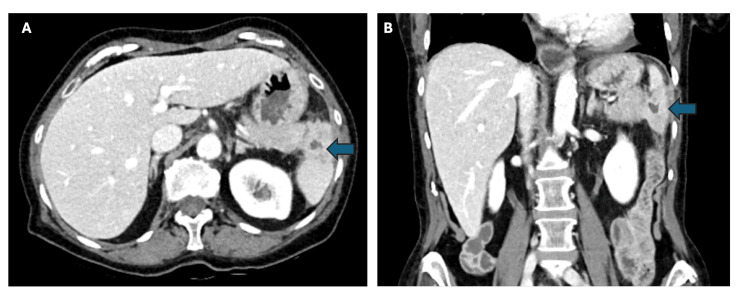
CT of the abdomen and pelvis with contrast CT of the abdomen and pelvis with contrast showing splenic fluid collection in the axial (A) and coronal (B) views status post drainage and antibiotics. Fluid collection is denoted by blue arrows​.
